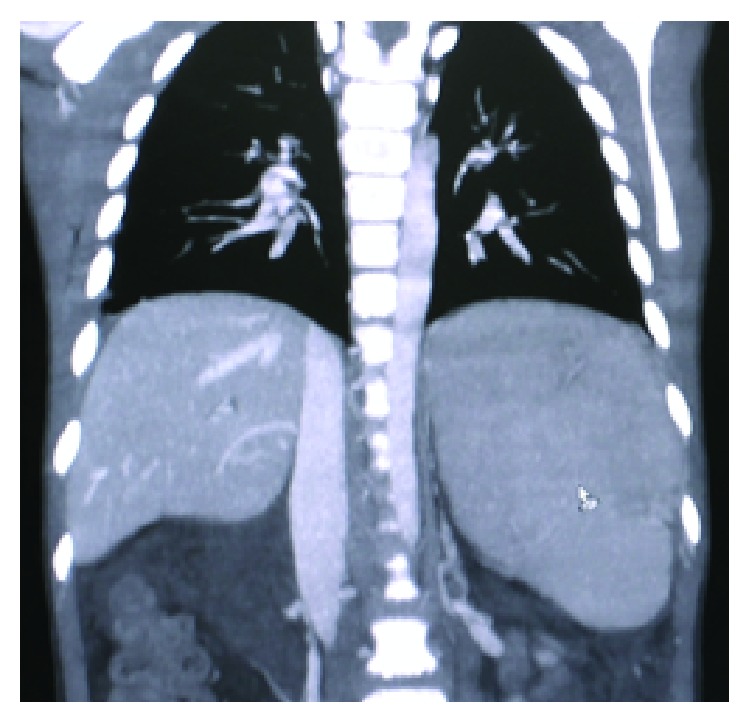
Patient's CT angiography of the abdomen revealed splenomegaly.
